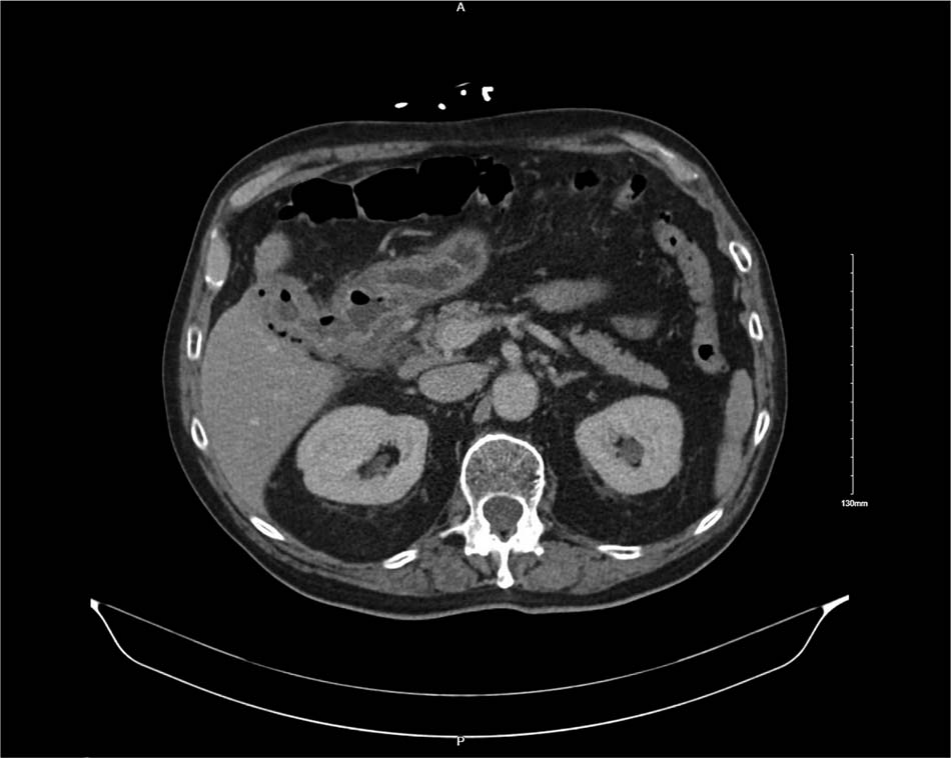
CT image showing cholocystoduodenal fistula at initial presentation.
